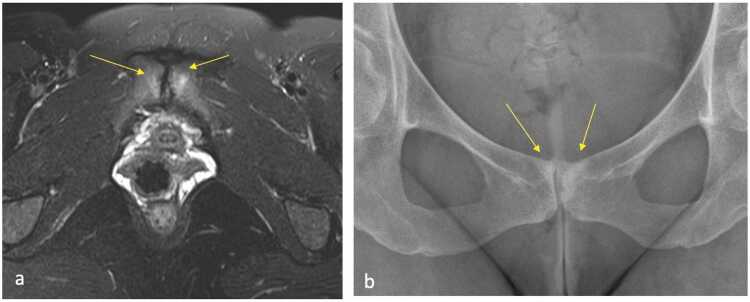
a) Axial T2 FS MRI performed 4 weeks after birth demonstrates bone marrow oedema of the pubic bone b) radiograph 1 year after birth demonstrates sclerosis consistent with osteitis pubis. DIAGNOSIS: Pubic bone marrow oedema.
